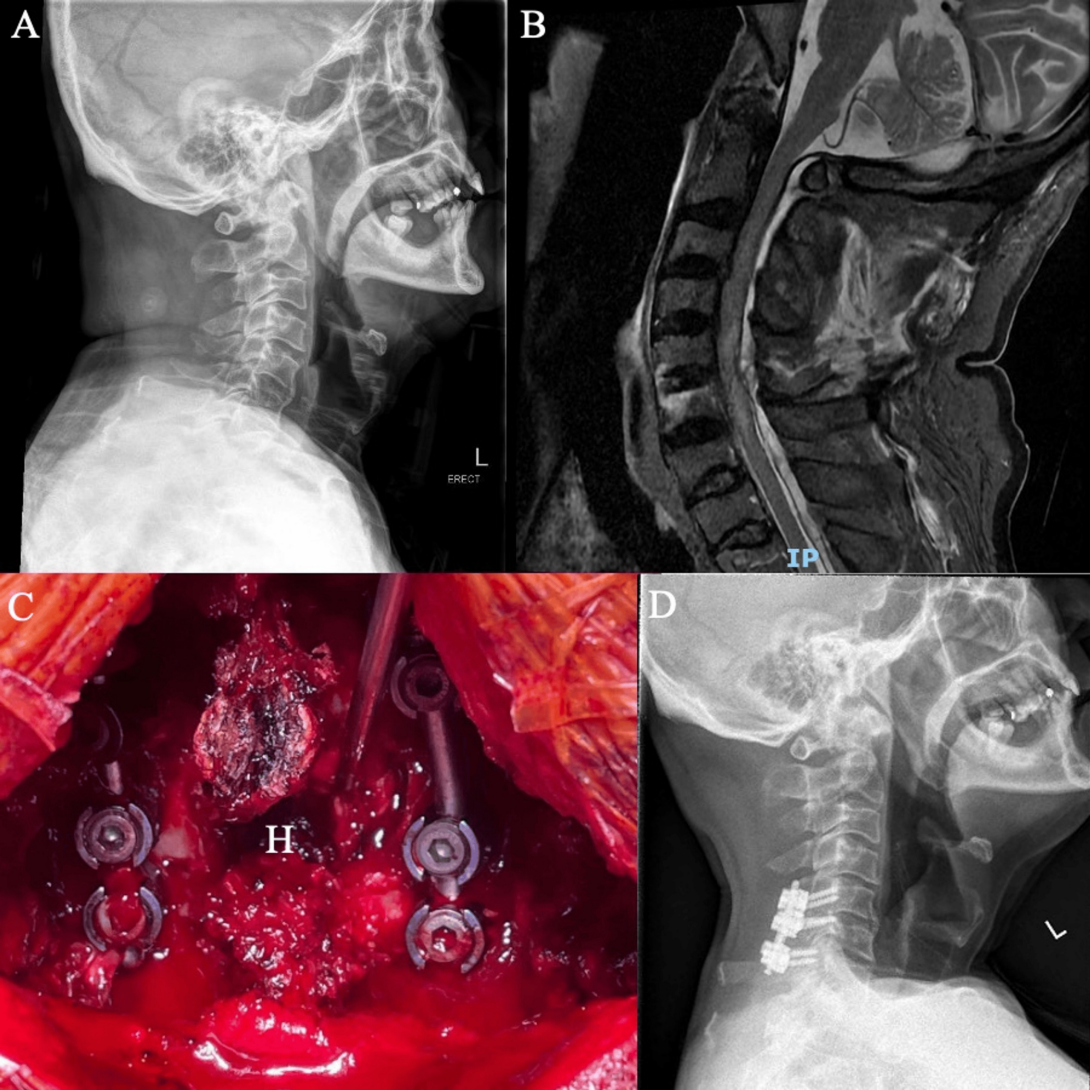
Case 1 (A) Lateral X-ray of the cervical spine showing minimally displaced vertebral body fractures involving the C5 and C6 levels. (B) MRI of the cervical spine (MRI-CS) showing a large epidural hematoma spanning C1 to T2 with cord edema from C4 to C6. (C) Intraoperative photograph with hematoma shown (labeled “H”). (D) Postoperative lateral XR cervical spine showing stable implants
